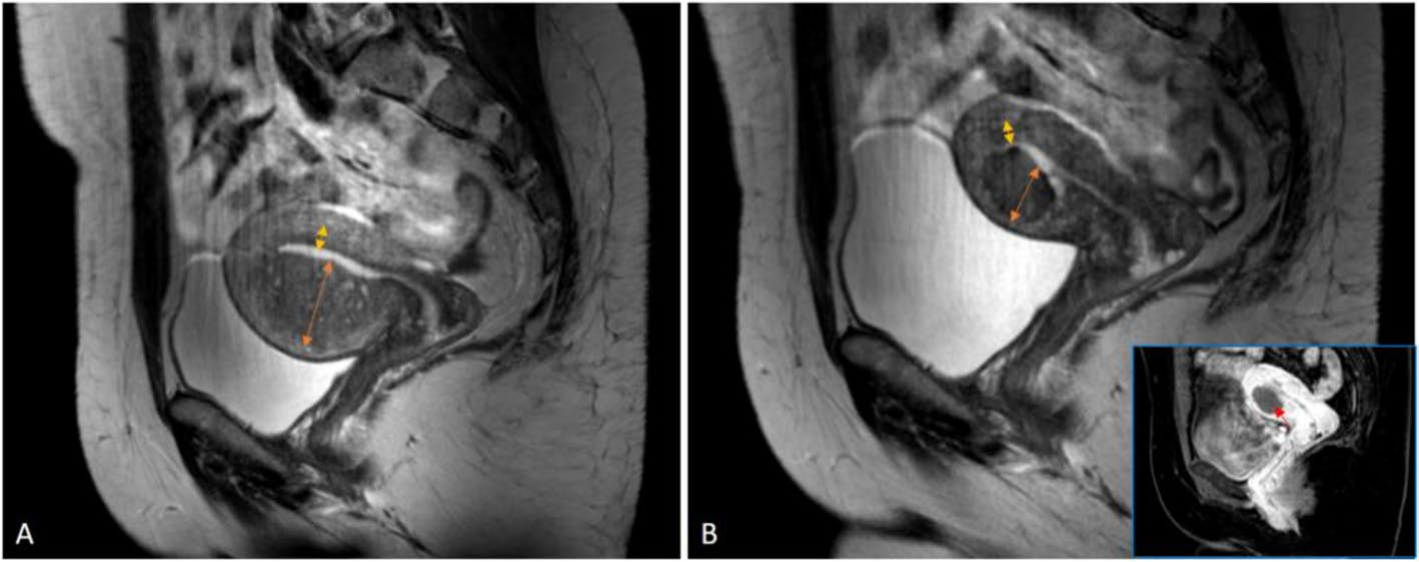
Preprocedural (A) and postprocedural (B) sagittal T2W images of a 49-year-old patient. The postprocedural examination was obtained 6 months after the UAE. Inserted sagittal contrast-enhanced T1-weighted image shows a necrotic area without contrast enhancement (red arrow on inserted image). JZmax (marked by an orange arrow on both images) was 35 mm before, and 21 mm after UAE. The minimal junctional zone thickness (JZmin) is marked by a yellow arrow on both images. JZdiff (JZmax-JZmin) was 33 mm before, and 16 mm after UAE. JZratio (not marked on images) was 0.88 and 0.84 before and after UAE, respectively. Uterus volume (not marked on the images) was 182 cm3 and 90 cm3 before and after UAE, respectively
